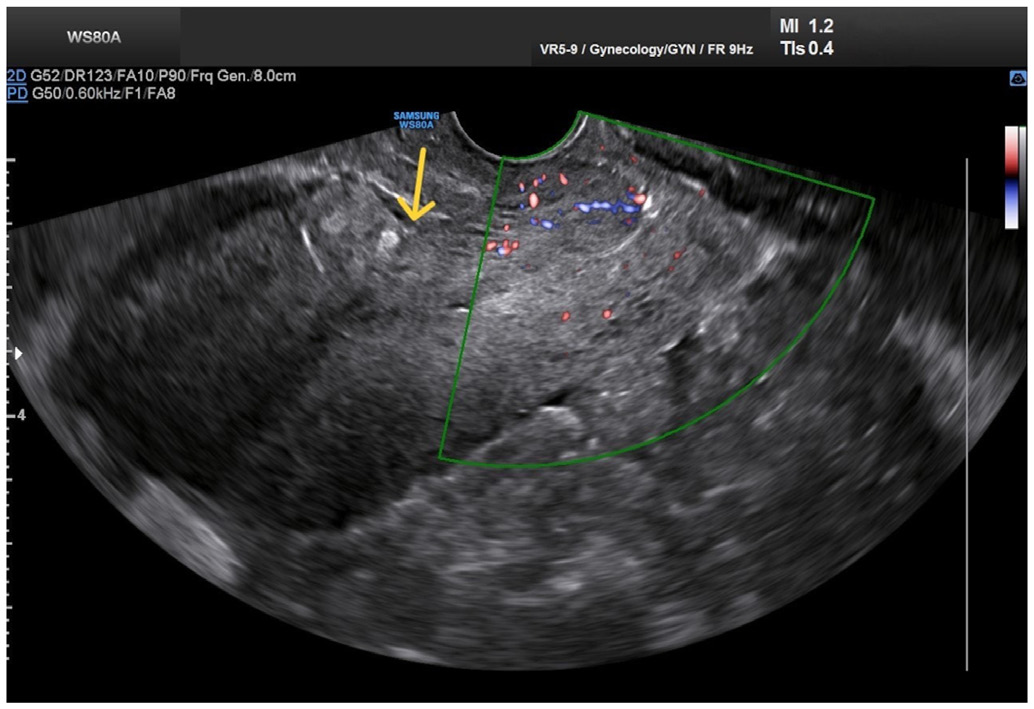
Transvaginal Doppler ultrasound imaging of the cervix 20 days after fetal loss: The findings are normal, with the cesarean scar healing site in the uterus (yellow arrow) appearing intact and without abnormalities.
